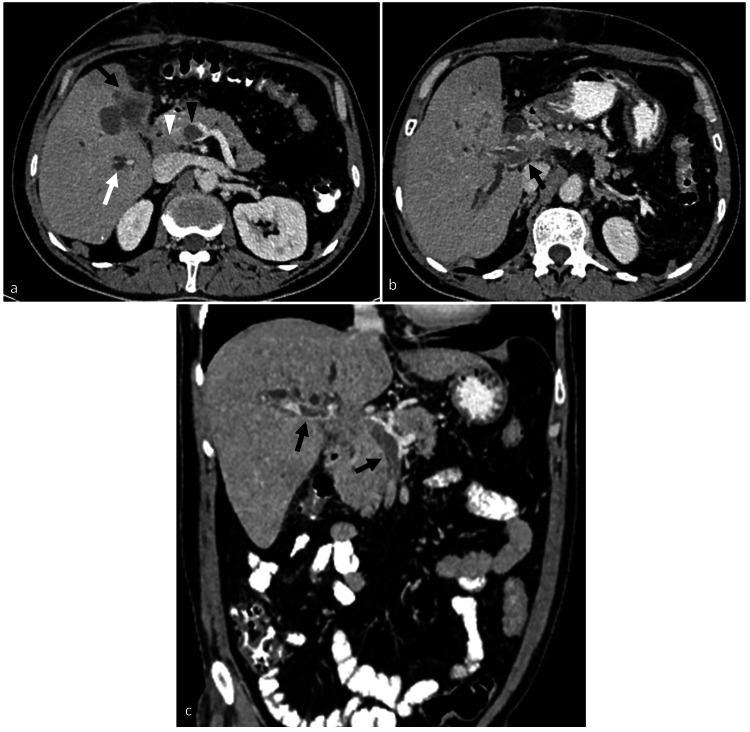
CT images showing the extension of tumor thrombus: (a) axial contrast-enhanced CT image obtained two weeks after the PET-CT shows the heterogeneously enhancing mass in the gall bladder fossa (black arrow), infiltrating the porta hepatis and adjacent liver parenchyma. Intrahepatic biliary radical dilation (white arrow), periportal lymphadenopathy (white arrowhead), and non-enhancing bland thrombus in the superior mesenteric vein (black arrowhead) are seen. (b) Axial contrast-enhanced CT image shows that the main portal vein is expanded with an enhancing filling defect within (arrow), suggesting tumor thrombus. (c) Coronal reformatted contrast-enhanced CT image shows a non-enhancing bland thrombus within the right portal vein and the superior mesenteric vein (arrows)
